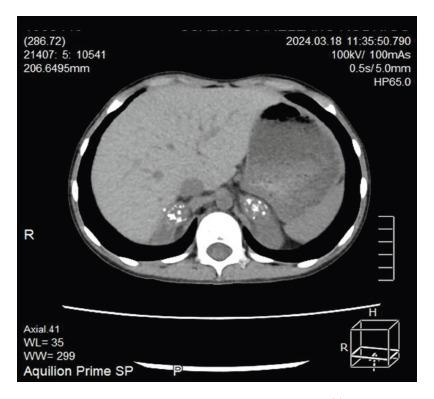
Bilateral adrenal calcifications on abdominal CT scan without contrast.
